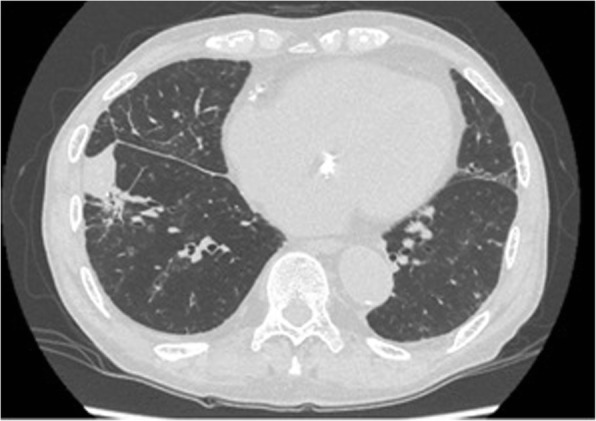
Chest CT scan 8 months after TAVI. The nodule in the right lower lobe grew larger
